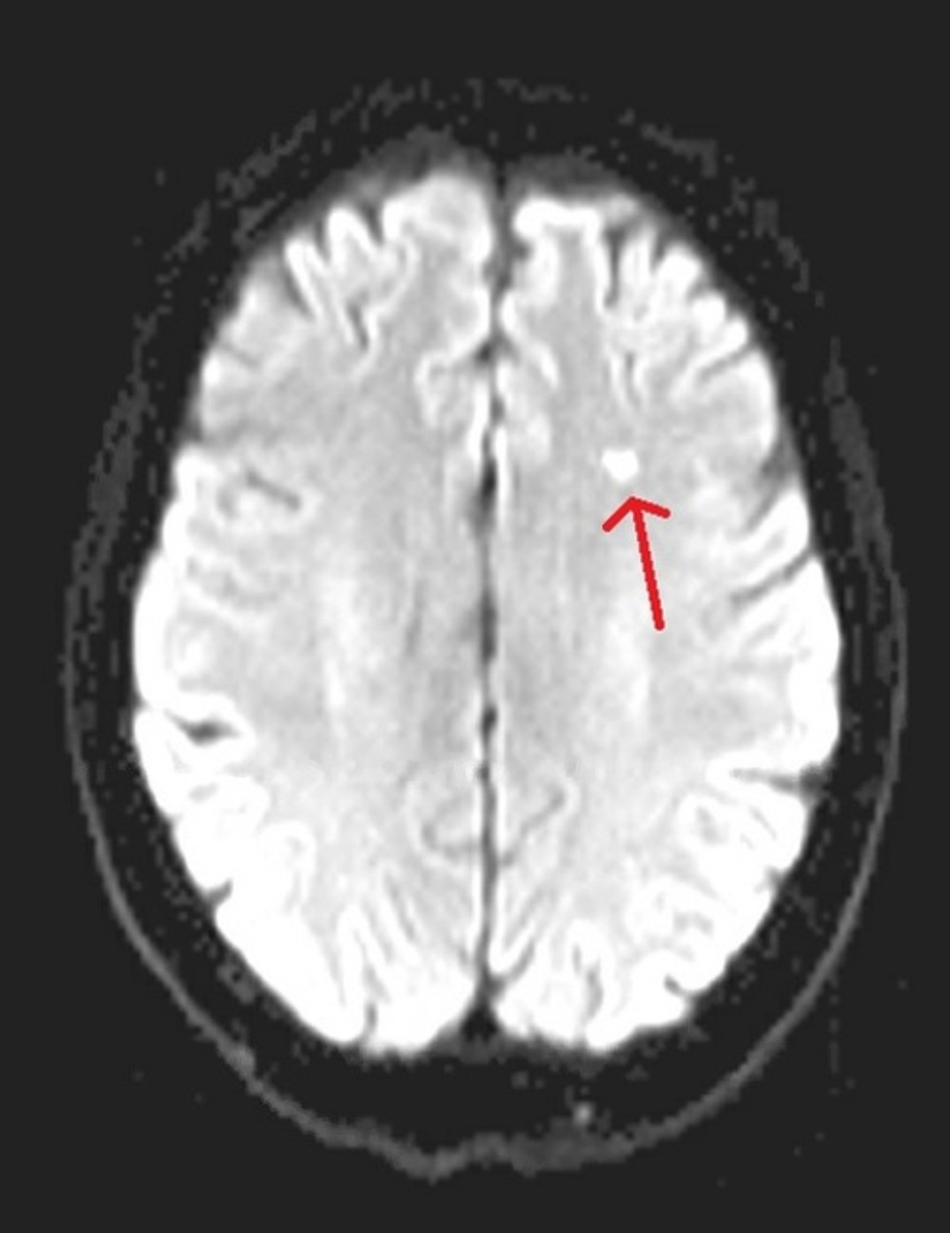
MRI of the brain Arrow depicts ischemic infarct in the left frontal lobe.
